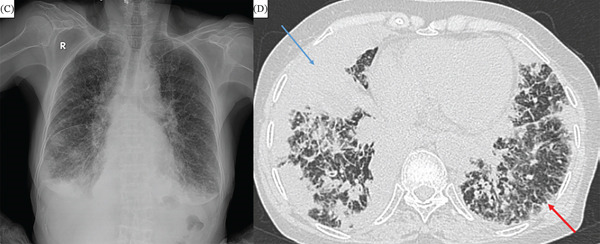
Diffuse alveolar septal amyloidosis. (C) Chest x‐ray revealing bilateral reticular opacities and infiltration with peripheral and lower lobe predominance. (D) Axial chest CT of the same patient demonstrating corresponding consolidation (blue arrow) and interlobular septal thickening (red arrow).
